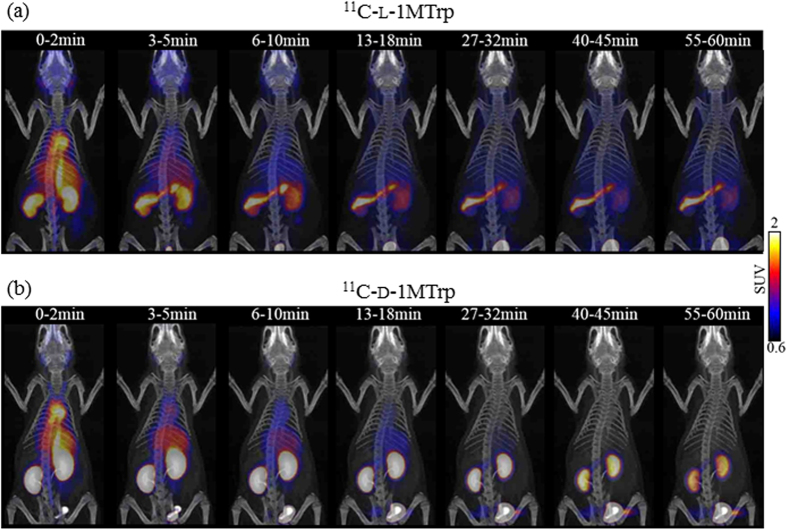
Representative pharmacokinetic images of the two isomers. (a) Three-dimensional PET/CT images at different time points after ^11^C-l-1MTrp injection. (b) Three-dimensional PET/CT images at different time points after ^11^C-d-1MTrp injection.
